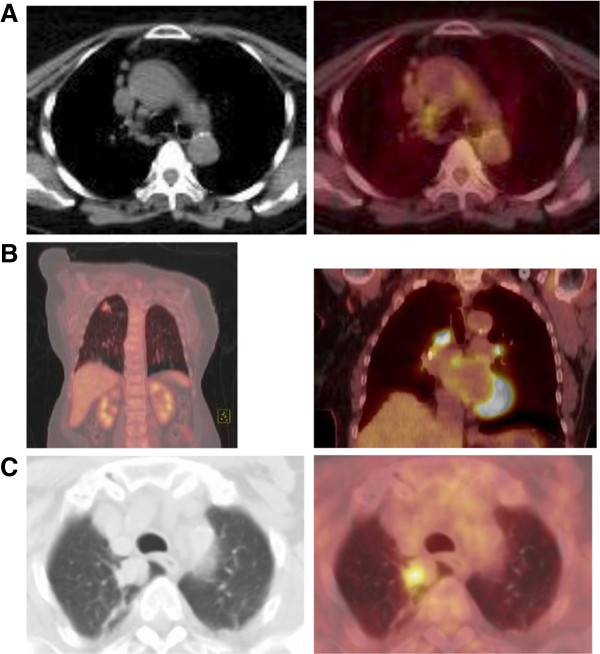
Representative FDG-PET/CT images. A. Case 1: axial CT (left) and fused FDG-PET/CT, with abnormal FDG uptake in the mediastinal lymph nodes. B. Case 2: fused FDG-PET/CT highlighting abnormal uptake in the right upper lobe nodule and more intense uptake in mediastinal lymph nodes. C. Case 3: axial CT (left) and fused FDG-PET/CT (right) with abnormal uptake in the right upper lobe nodule.
